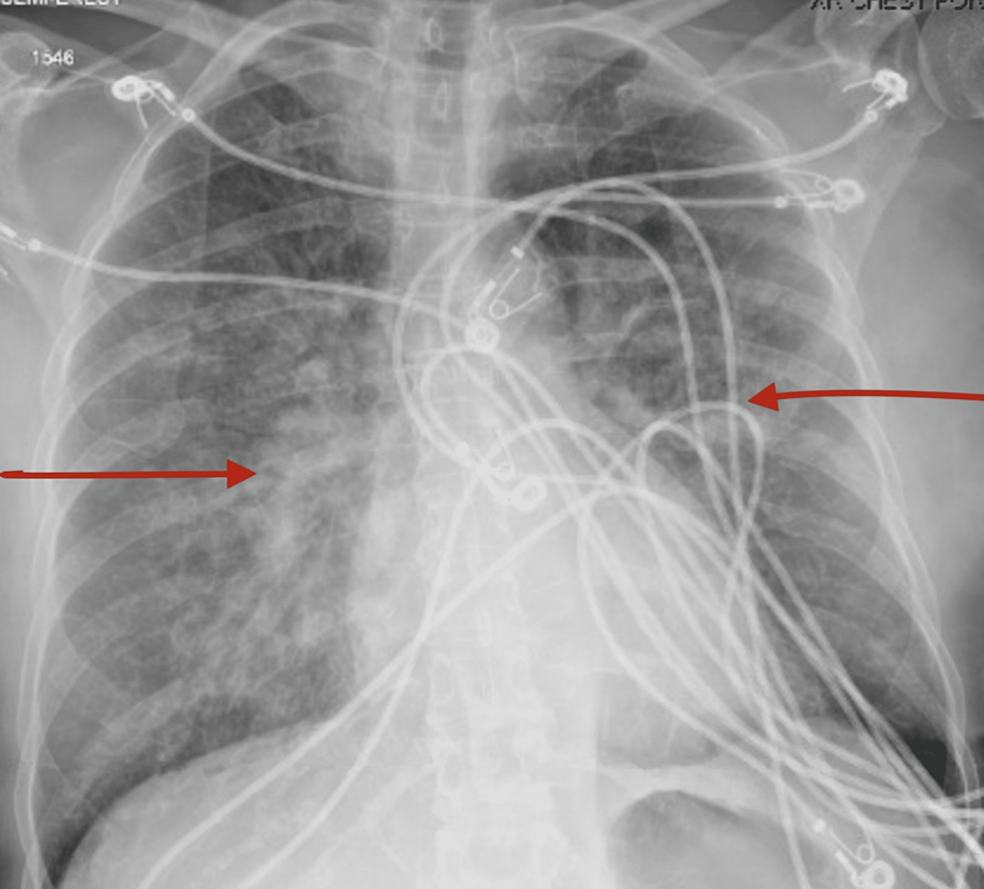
Chest X-ray showing bilateral pulmonary infiltrates (see red arrows).
